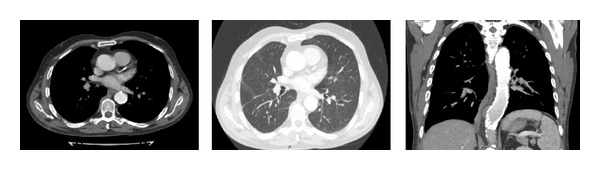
A follow-up CT angiography, three months later showed a smooth and patent endograft with no migration or endoleak. The lung is fully re-expanded.
